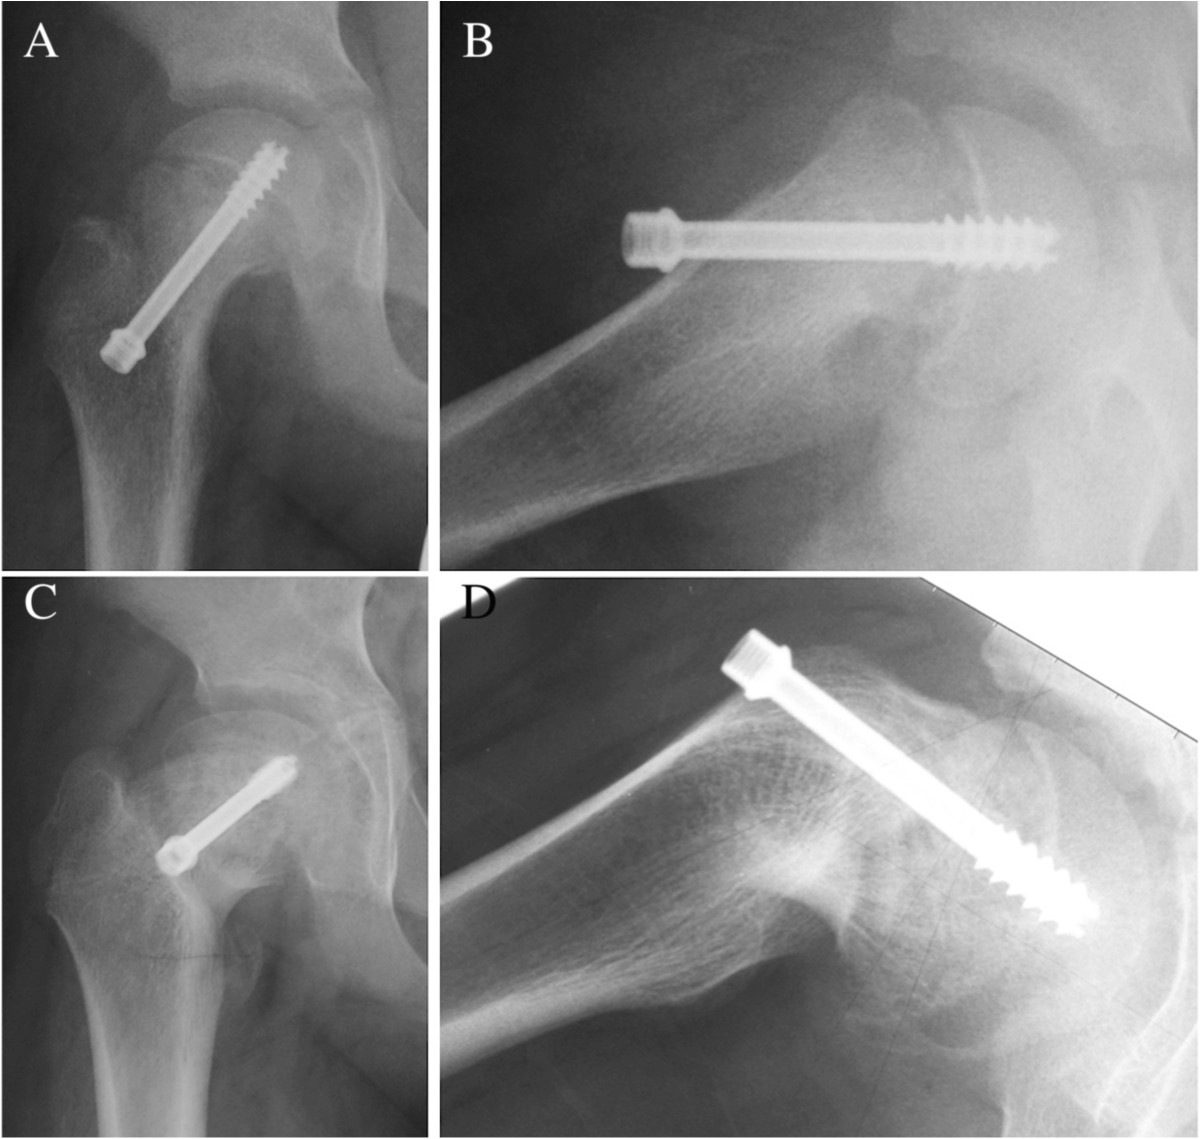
Radiographs of the right hip in a 10-year-old boy with SCFE. Anteroposterior (A) and lateral (B) radiographs immediately after ISF for chronic and stable type hips at 37 degrees of PTA. A single screw was inserted from the medial point to the center of the epiphysis perpendicularly. Anteroposterior (C) and lateral (D) radiographs at physeal closure. PTA had progressed to 56 degrees 18 months after surgery due to excessive retroversion of the femoral neck.
